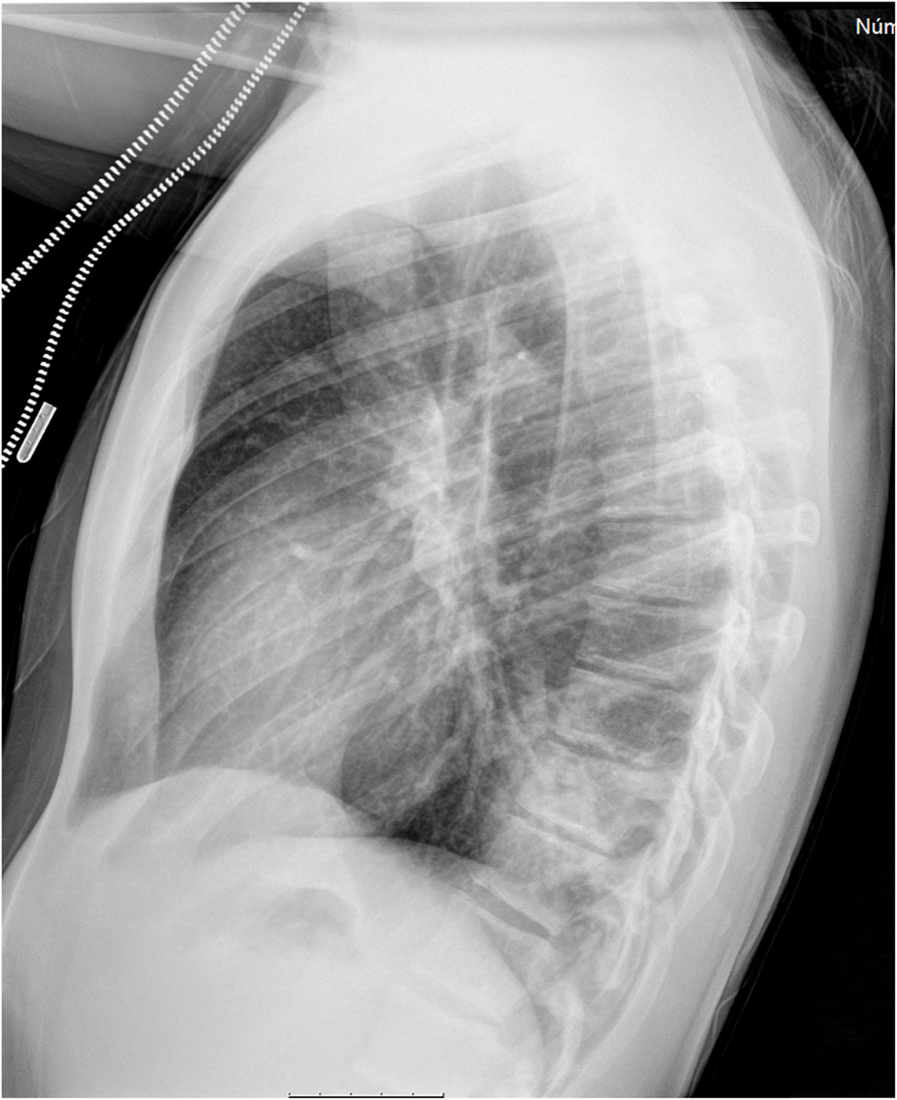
Left lateral chest x-ray performed on day 14 showing an improvement of the opacity
